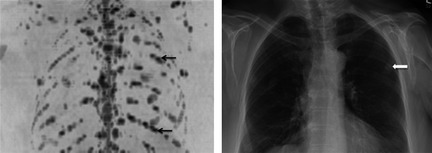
Diffusion weighted magnetic resonance imaging (DW‐MRI) is highly sensitive for detection of focal rib lesions. Left: Inverted greyscale b900 whole body DW‐MRI Maximum Intensity Projection of the chest in a 53‐year‐old female with multiple myeloma shows numerous sites of disease in the ribs (examples indicated by arrows). Right: A chest radiograph in the same patient shows rib fractures (block arrow) but no lytic lesions.
